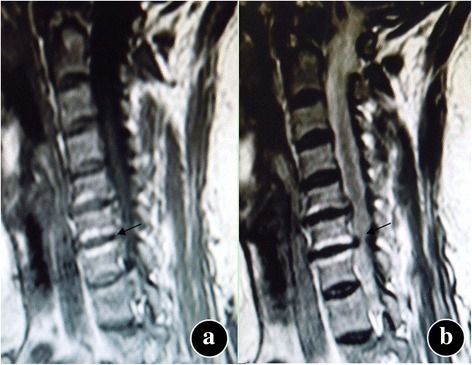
Modic type I changes. Hemispheric low signal intensity on T1-weighted (a) and high signal intensity on T2-weighted (b) images are noted on both sides of the endplate at the C6–7 level in the cervical spine
